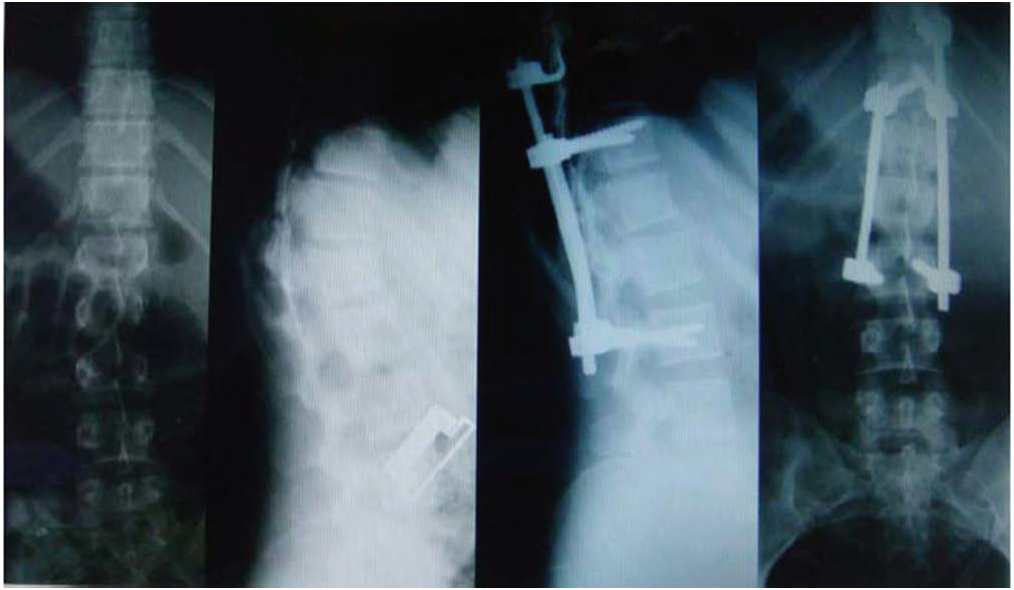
Initial radiographs. The initial trauma caused L1-L2 flexion-distraction injury. The patient was first treated by posterior spinal fusion and instrumentation from T11 to L3.
